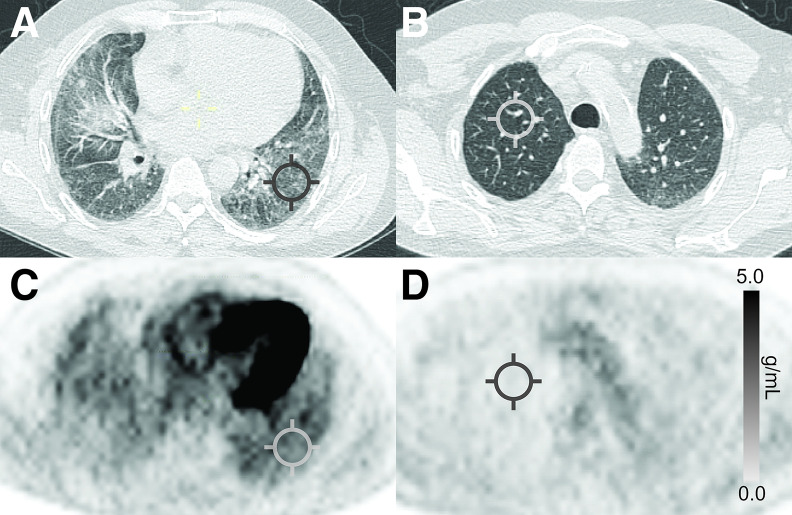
Axial CT and corresponding [^18^F]FDG PET emission scans of patient with nonspecific interstitial pneumonia. CT placement of regions of interest on morphologically abnormal (A) and normal (B) lung. Corresponding regions of interest on [^18^F]FDG PET emission scan for determining SUV_max_ (C) and SUV_min _(D).
